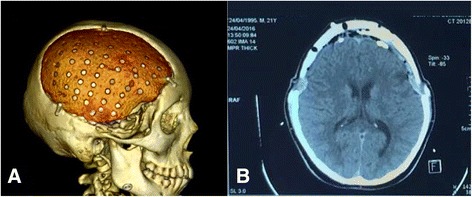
A Case Report 1 postoperative CT. Notice the coaptation of the prosthesis to the bone defect. B Case Report 2 postoperative CT. Notice the expansion of the frontal lobes on these axial plane images which were collapsed preoperatively
